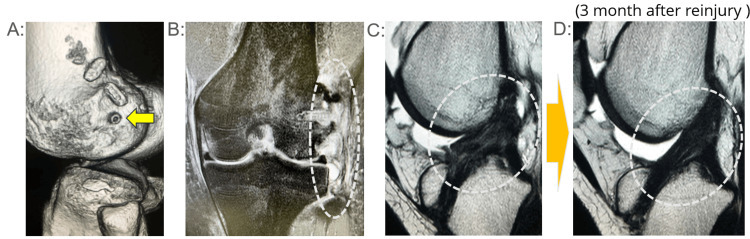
Case 4 (CT・MRI) (A) Postoperative computed tomography (CT) image showing the location of the femoral tunnel for the lateral extra-articular tenodesis (LET), indicated by the yellow arrow. (B) Magnetic resonance imaging (MRI) reveals extensive soft tissue damage extending from the region surrounding the tenodesis screw used for LET fixation to the entire iliotibial band (ITB). (C) The midsubstance of the reconstructed anterior cruciate ligament (ACL) demonstrates evident structural injury; however, partial fiber continuity is still preserved. (D) Follow-up MRI obtained three months after reinjury shows improved continuity of the ACL midsubstance, indicating spontaneous healing
